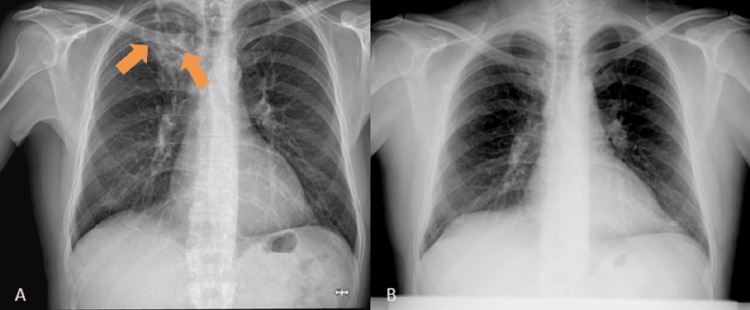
Images of the chest radiographs (A) Chest radiography taken on the day of admission to the emergency department, showing heterogeneous rounded hypertransparency of important dimensions at the apex of the right lung (orange arrows). (B) Chest radiography performed 10 years before, showing no abnormalities
